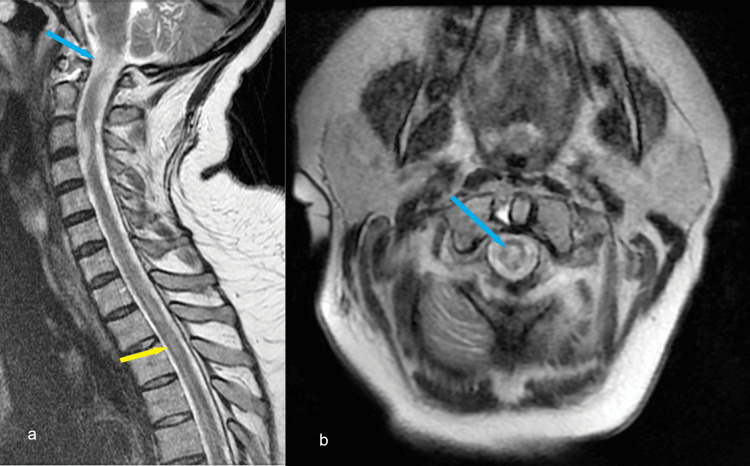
a: sagittal T2 MRI showing new C1-C2 (blue arrow) and T2-T3 intramedullary T2 hyperintense areas (yellow arrow); b: axial T2 sequence of the C1-C2 intramedullary lesion (blue arrow)
